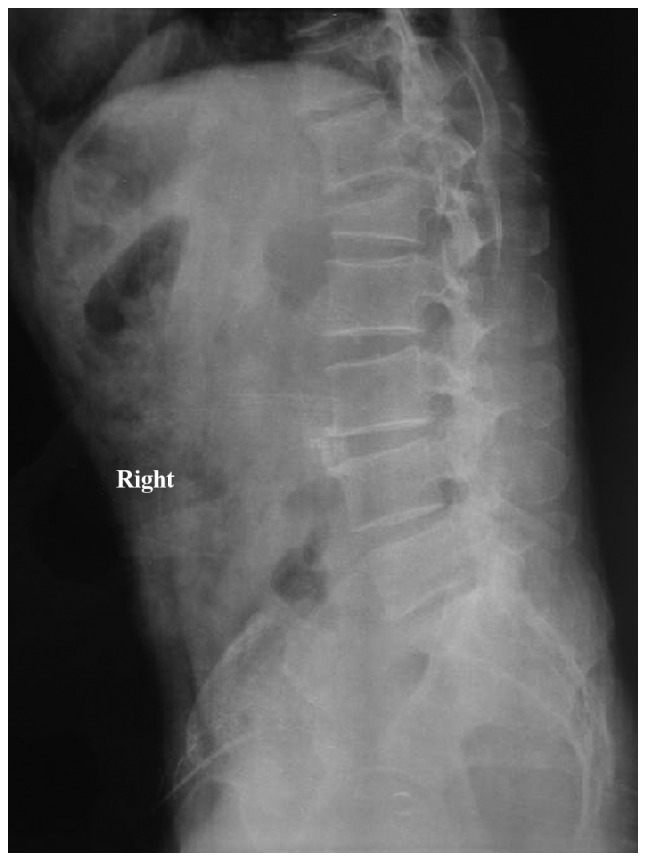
Preoperative X-ray film of a 45-year-old male patient showed a fracture at L1. The height of L1 was compressed to a half of that of a normal vertebral body.
